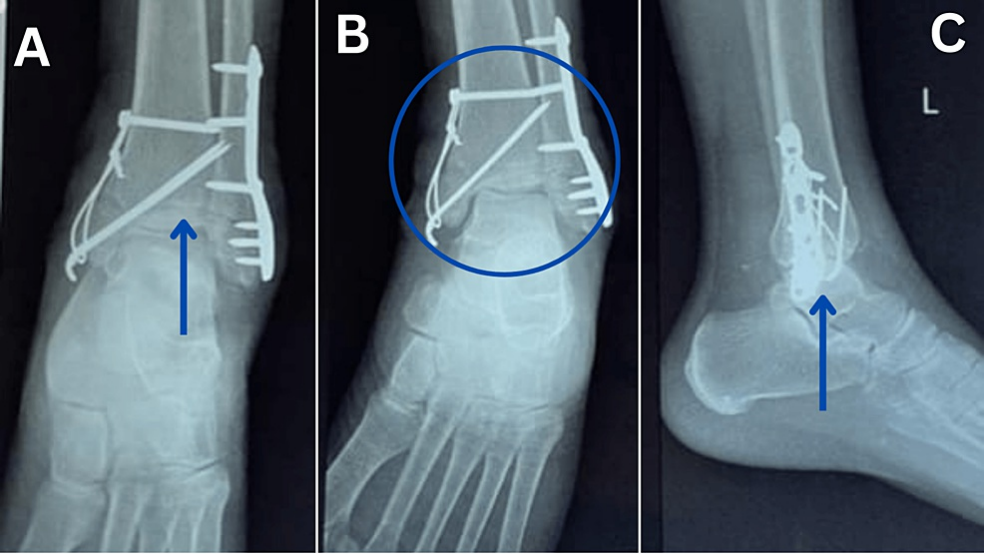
X-ray of post-operative medial and lateral malleolus fracture (left side) Figure 2A and 2B: Arrow and circle show ORIF done with nailing and plating in (anterior-posterior view) Figure 2C: Arrow show ORIF done with nailing and plating (lateral view) ORIF: Open reduction and internal fixation
